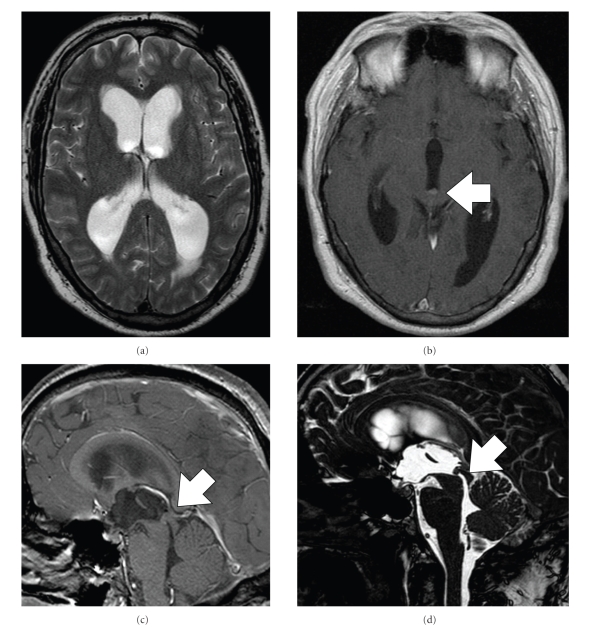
(a) T2-weighted MRI showing marked hydrocephalus, T1-weighted contrast enhanced MRI reveals a small lesion tumor at the cranial end of the aqueduct in axial (b) and sagittal view (c) and TRUFI sequences (sagittal thin T2-weighted images, (d)).
